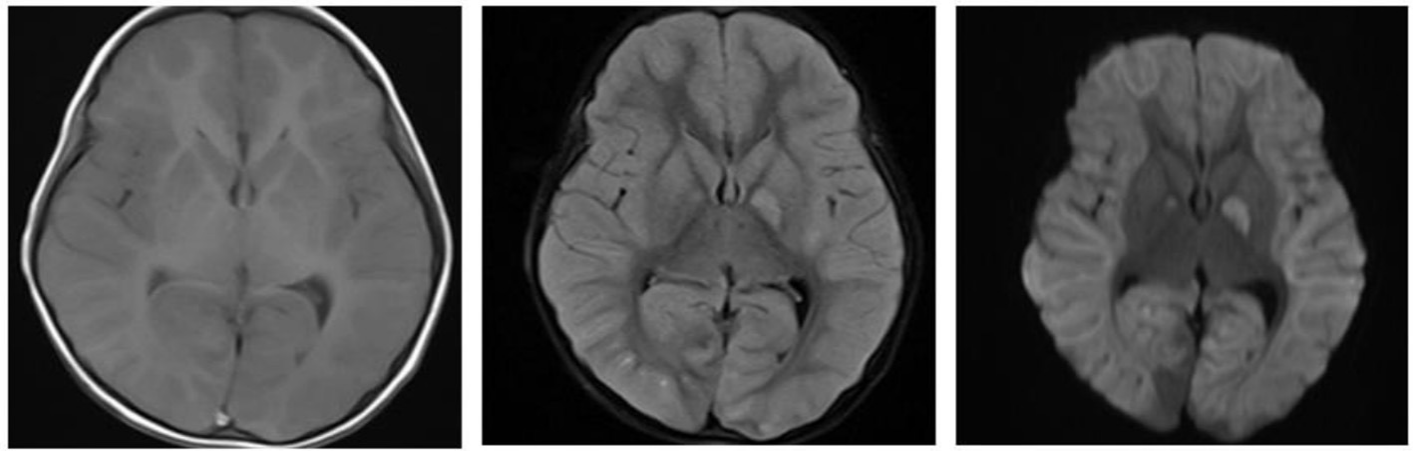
Cranial MRI on 10th day.
